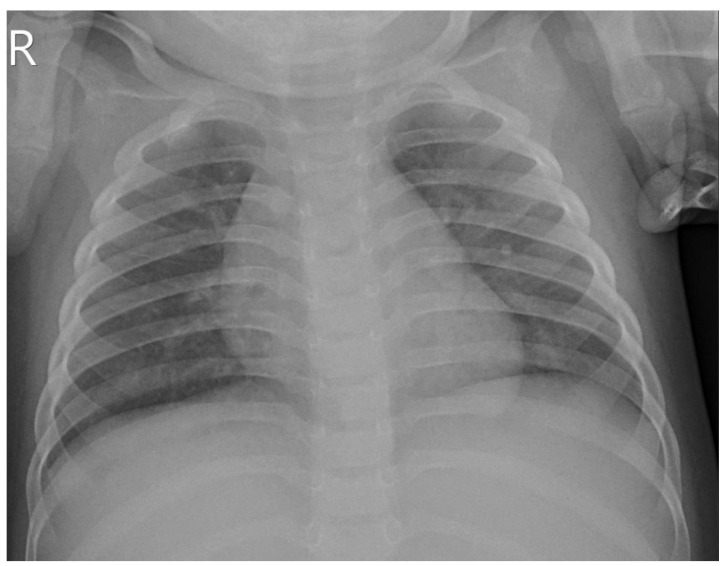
Chest radiograph at discharge demonstrating resolution of pulmonary infiltrates.
